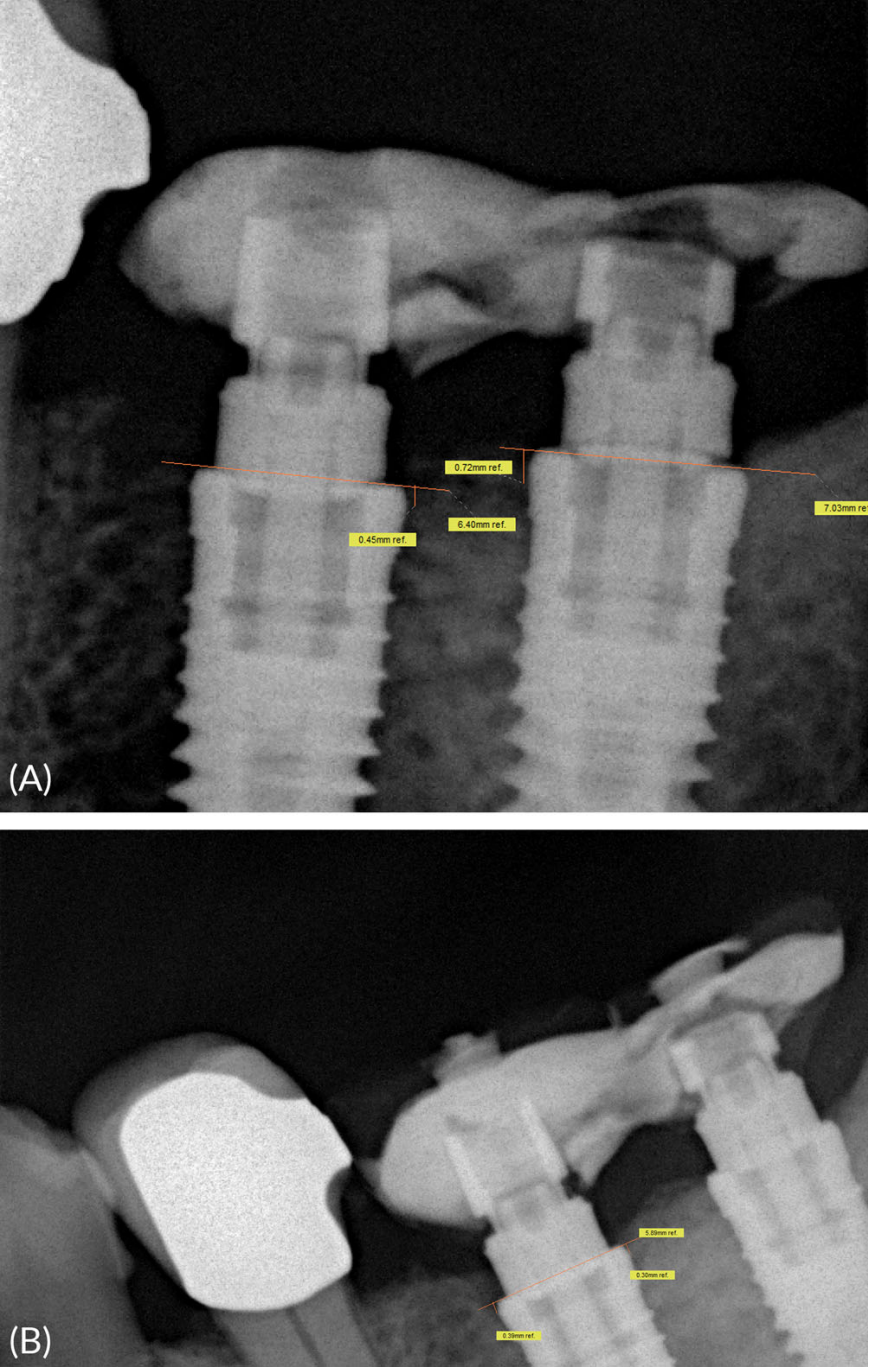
Marginal bone defects as measured in the present study, based on radiographs and using a horizontal reference line at baseline and 36 months post op
